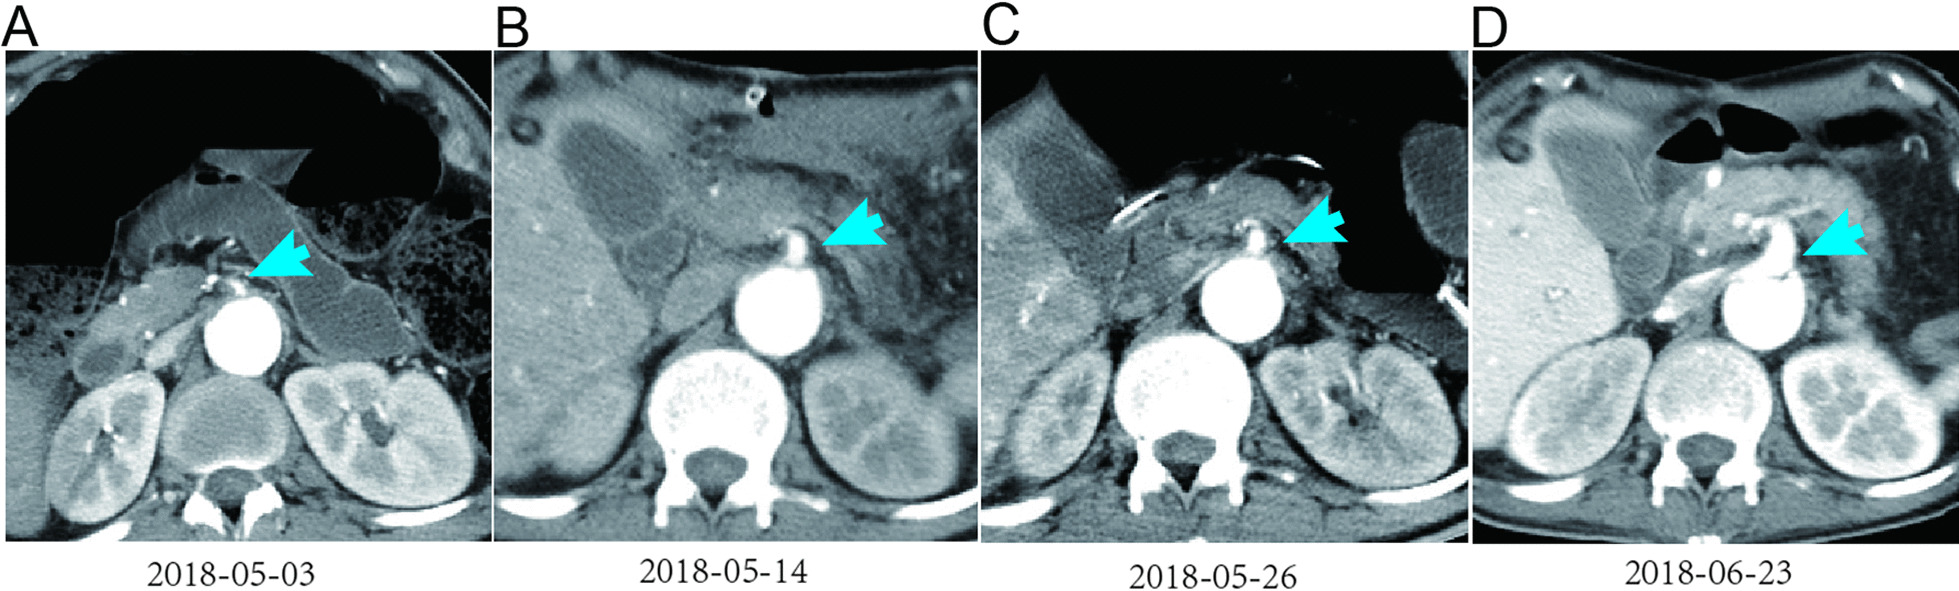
Abdominal computed tomography of the patient with aortic dissection after the intracavitary aortic repair D showed that the diameter of the SMA increased significantly compared to that before the operation (A–C)
